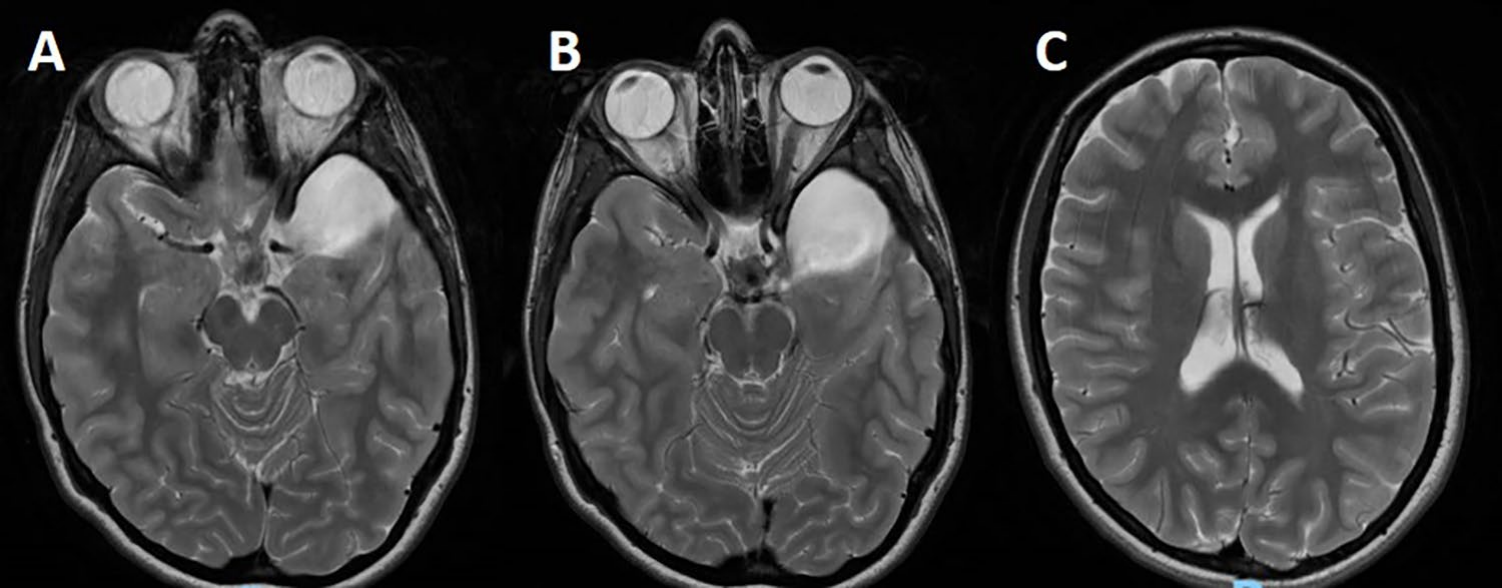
MRI, realized about 2 years from the onset of the clinical history, showing a slight decrease of the cyst volume and the flow artifact indicating the fenestration (A, B). No recurrence of the hygroma is evident (C)
